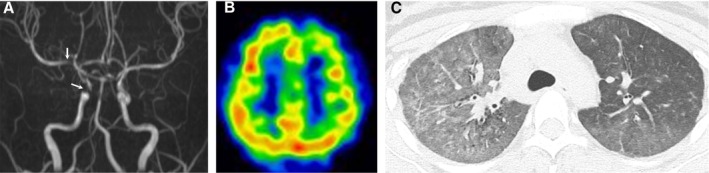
Two cases with SLE. (A, B) A 12‐year‐old girl with neuropsychiatric SLE presenting with severe headache. Conventional MRI showed no signal abnormalities on T1WI or T2WI (not shown). MR angiography (A) showed multifocal stenosis of the major intracranial arteries (arrows). 123I‐IMP brain perfusion SPECT (B) demonstrated multifocal decreased uptake in both cerebral hemispheres. (C) A 16‐year‐old girl with SLE complicated by diffuse alveolar hemorrhage. CT showed extensive, non‐segmental ground‐glass opacities with interlobular septal thickening in both lungs, more pronounced on the right.
